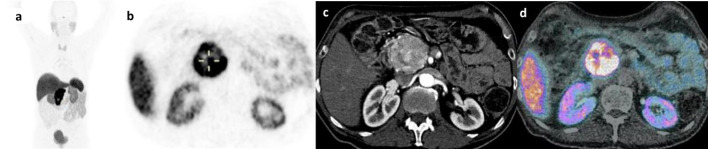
^68^Ga-DOTATATE PET/CT images showing intense uptake of a grade II pancreatic NET of the uncinate pancreatic process in a 73 year man with; a MIP image, b Axial PET slice, c Axial CE-CT slice and d fused axial PET/CT slice
